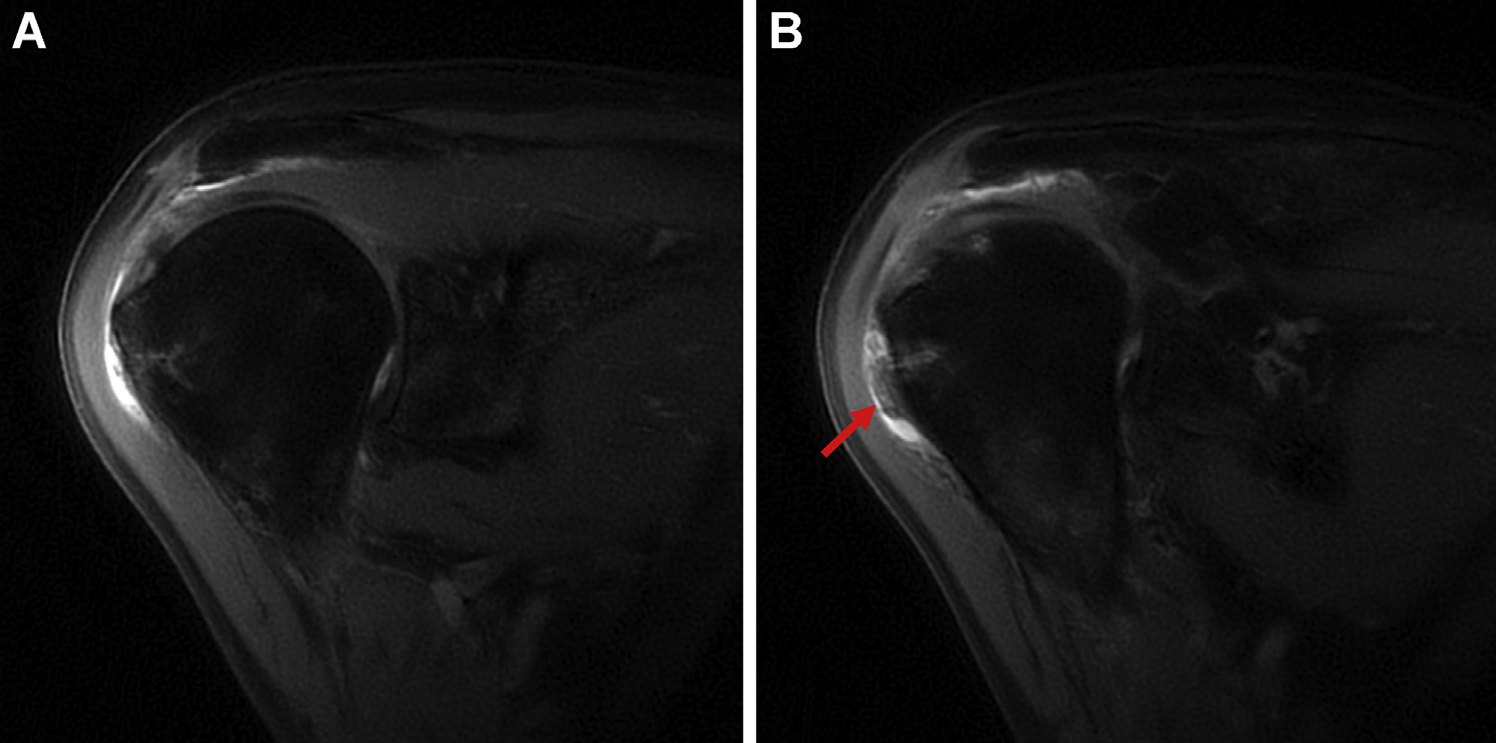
Case one follow-up - proton dense fat-saturated MRI; coronal oblique sections evidenced integration of the collagen scaffold, improvement in interstitial tearing of the rotator cuff, and a new reactive subacromial bursitis. There is also notable scar tissue in the lateral subdeltoid recess ().
